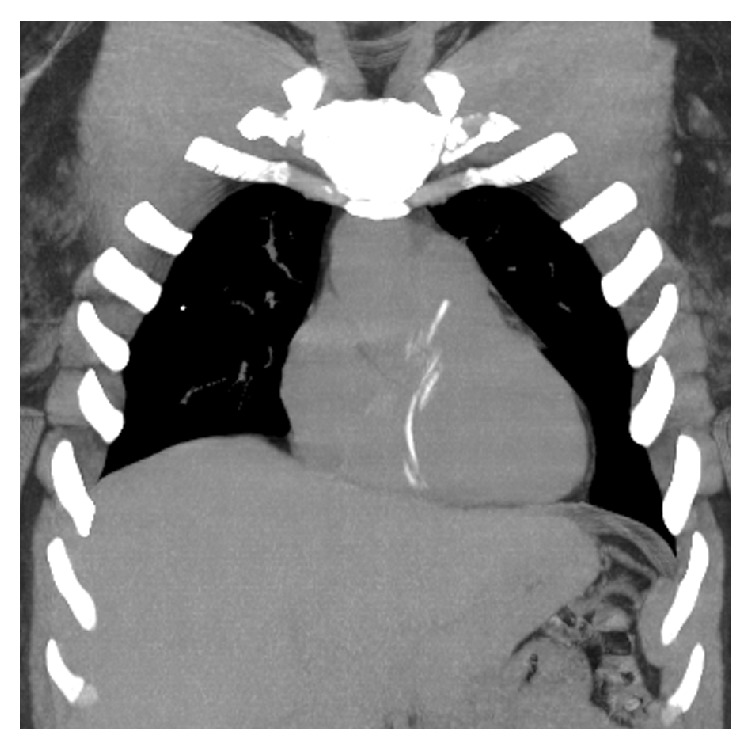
Coronal reformat noncontrast CT MIP image demonstrates the dislodged catheter extending from the right atrium through the right ventricle terminating in the pulmonary outflow tract.
